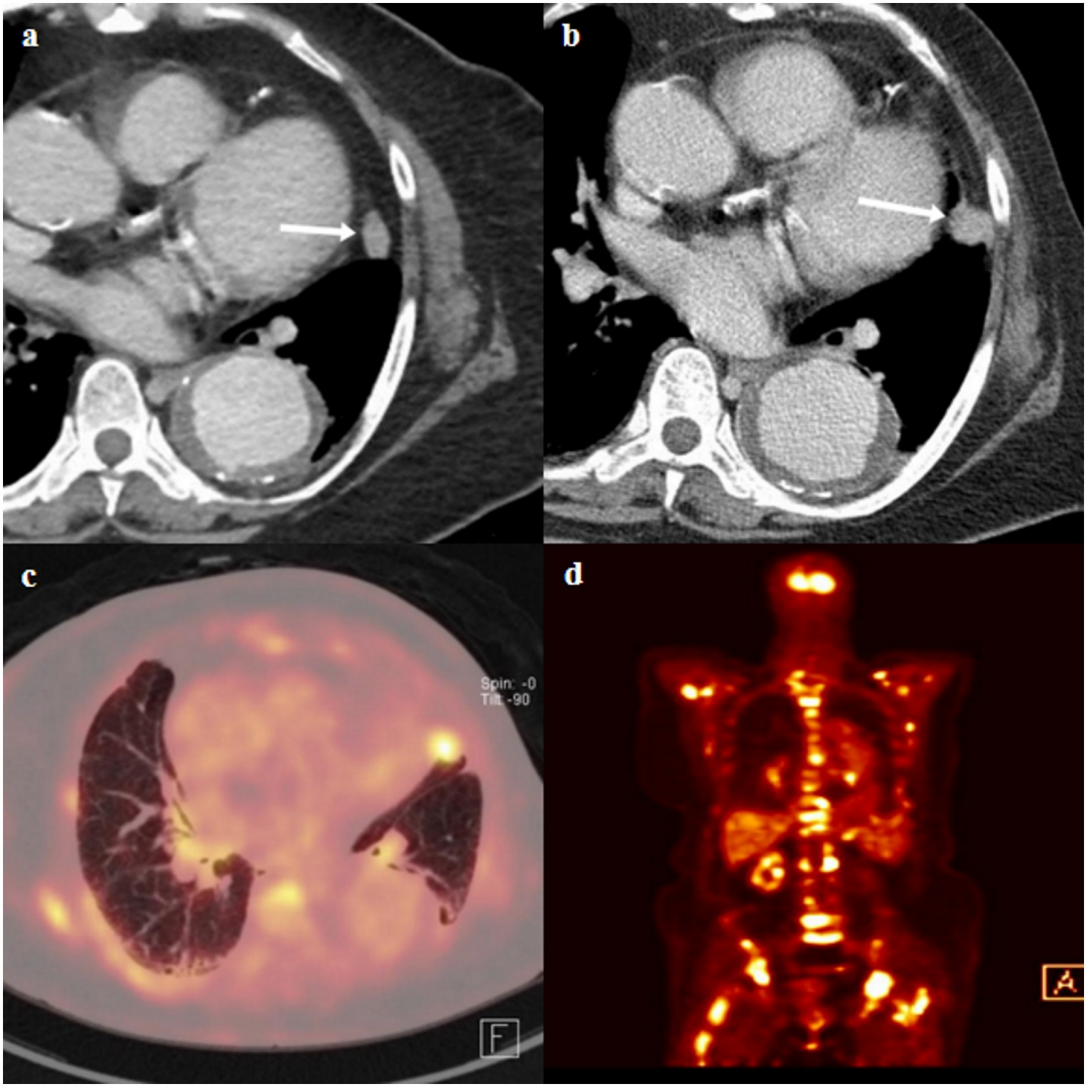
CT and fluorine 18 fluorodeoxygluocose (FDG) positron emission tomography (PET)/CT images of a true lung metastasis of a 71-year-old female with pathologic stage III breast cancer. (a) Staging chest-CT image shows an enhancing indeterminate nodule (arrow) in left upper lobe lingular segment. In addition, descending thoracic aortic aneurysm was incidentally detected. (b) A follow-up chest-CT image shows an interval growth of nodule (arrow). (c and d) Axial and coronal FDG PET/CT scans show FDG uptake in a growing nodule in the left upper lobe lingular segment (SUV_max_, 7.7) and multiple axial bony thorax (SUV_max_, 12.1). The bony lesion of the left ilium (not shown) was pathologically confirmed as metastasis after CT-guided biopsy. The nodule in the left upper lobe lingular segment was subsequently regarded as pulmonary metastasis.
